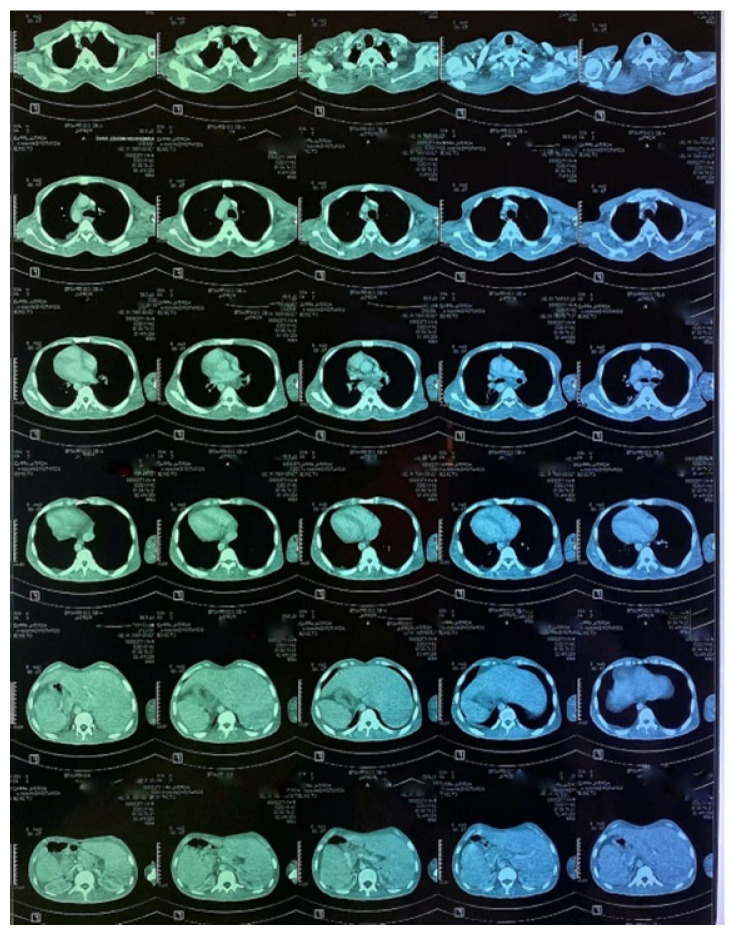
CT images of the thorax and abdomen:axial view.
